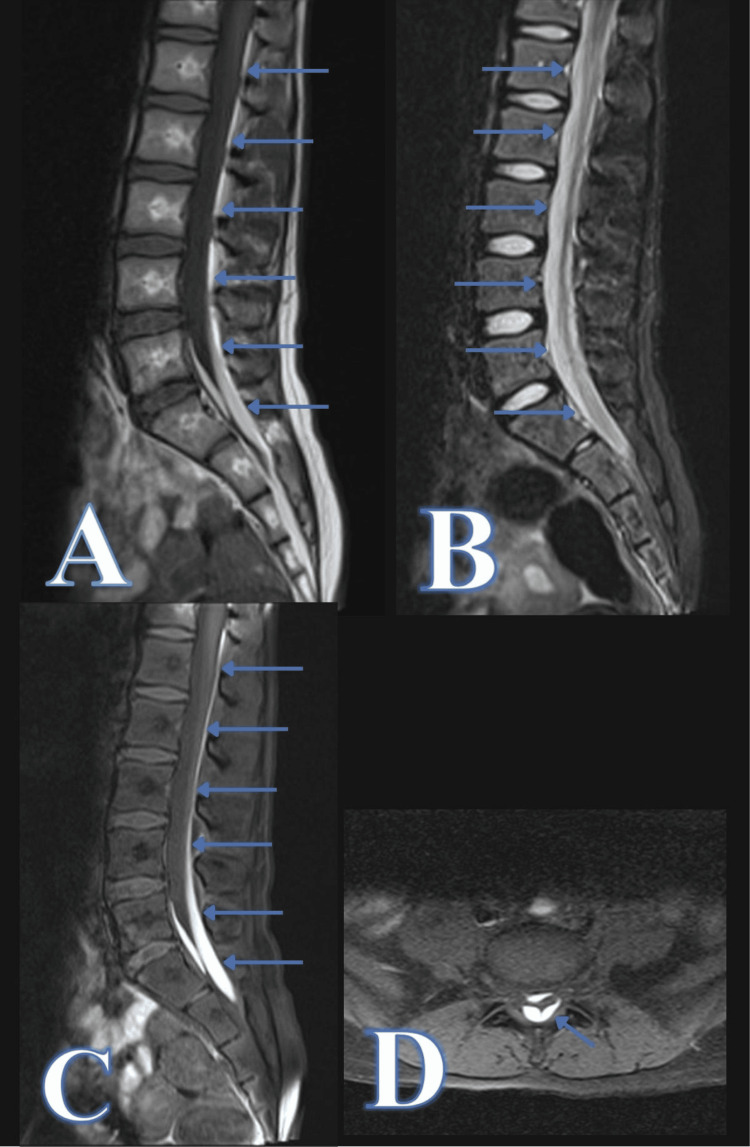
Diagnostic lumbo-sacral magnetic resonance imaging demonstrating a spinal subdural hematoma at L1-S2 levels. Sagittal T1 without fat suppression (A), sagittal short tau inversion recovery (STIR) (B), sagittal T1 with fat suppression (C), and axial T1 with fat suppression (D).
